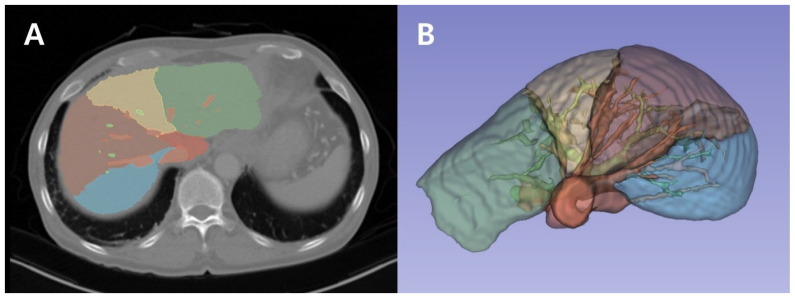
Liver segmentation using a 3D residual U-Net model. (A). Segmented liver from abdominal CT scan. Liver segments are color-coded to demonstrate regional anatomical structures, as viewed from the axial plane (horizontal cross-section). (B). 3D volumetric rendering of the liver. The liver lobes and associated vascular structures are segmented and color-coded to enhance clarity.
